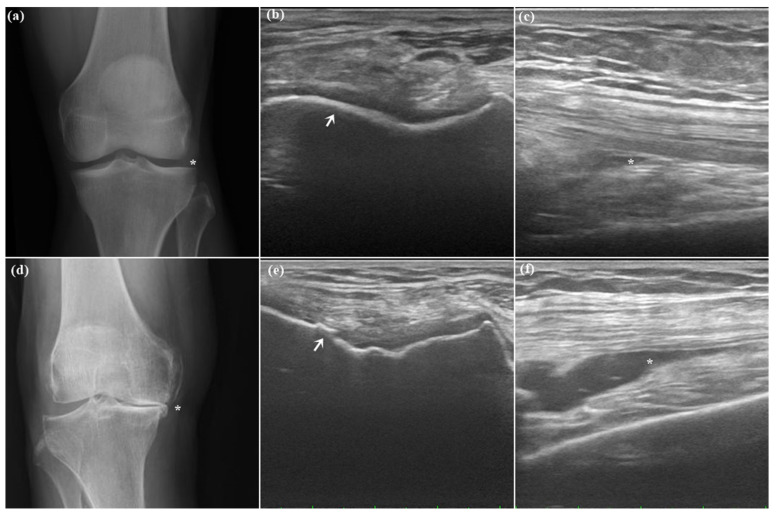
Knee X-ray (a,d) and ultrasound (b,c,e,f) in an individual with a healthy knee (a–c) and a patient with stage IV knee osteoarthritis (d–f). (a) Normal joint space without narrowing. (b) Normal hyaline cartilage (arrow). (c) Minimal joint effusion over SF (asterisk). (d) Large osteophytes, marked joint space narrowing, severe sclerosis, and definite bony deformity (asterisk). (e) Irregular hyaline cartilage (arrow). (f) Moderate effusion over SF (asterisk).
